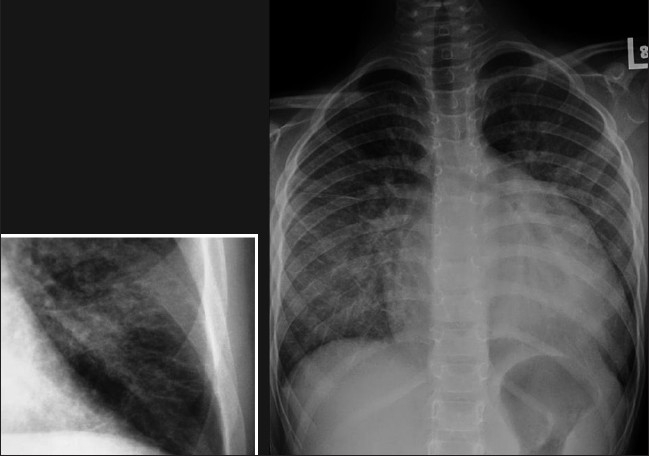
Frontal chest radiograph (right) showing features of interstitial pulmonary edema. Radiographic signs (shown in the figure) that suggest interstitial pulmonary edema include loss of definition of large pulmonary vessels, the appearances of septal lines, interlobar septal thickening and diffuse reticular pattern associated with cardiomegaly. Both Kerley's A and Kerley's B lines are seen. The magnified view of the left costophrenic angle is from another patient, depicting Kerley's B lines (left)
